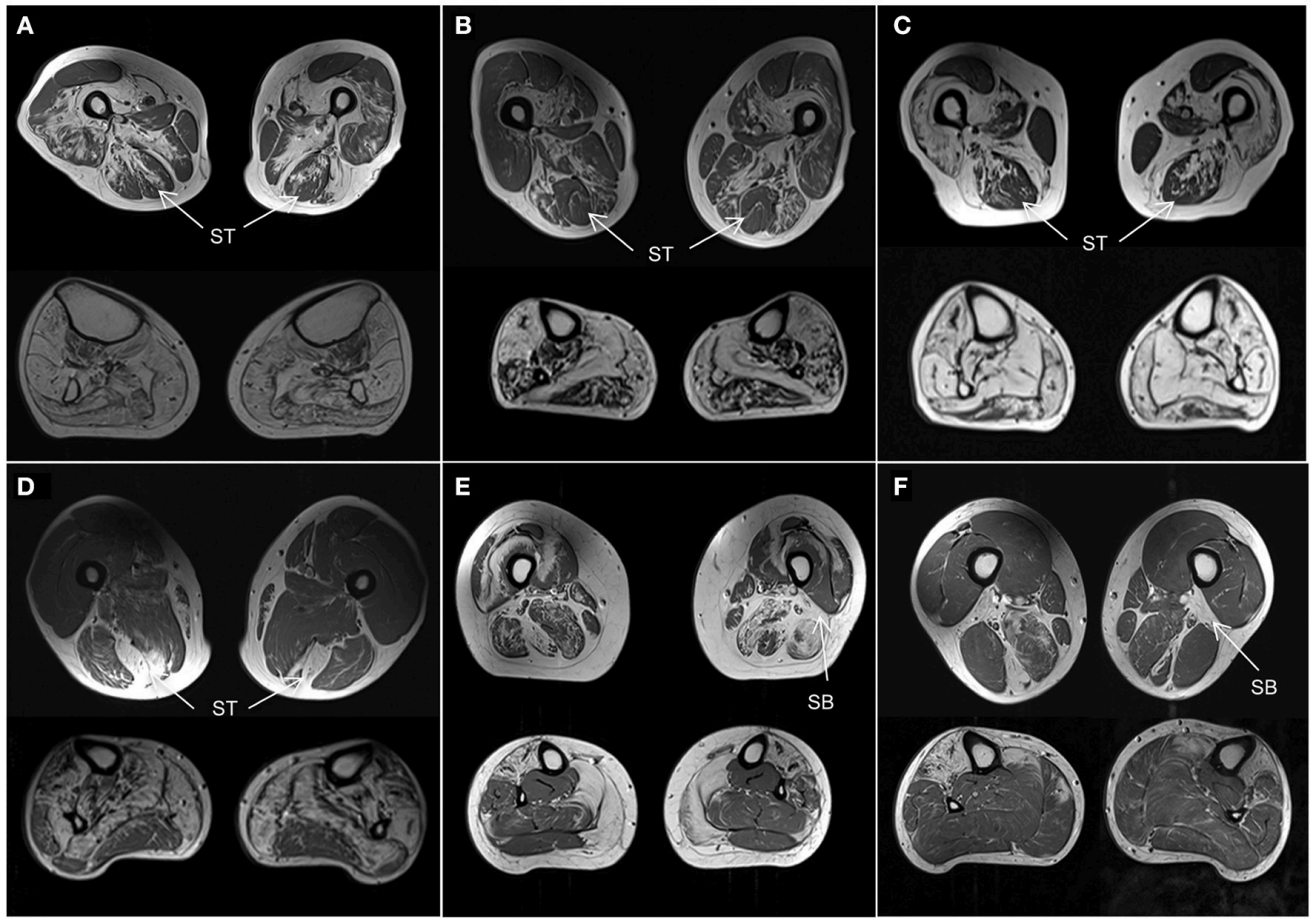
Muscle MRI patterns. Axial T1-weighted images at mid-thigh and mid-calf level in selected subjects. (A–C) Patients with genetically confirmed *MYOT* mutations all correctly identified. They are all characterized by a relative sparing of the semitendinosus (ST) muscle. The vastus intermedius and sartorius muscles are more affected than the gracilis muscle. All calf muscles can be affected; the gastrocnemius lateralis muscle is usually less affected than the medialis. (D) Patient with *TTN* (ID18) mutation. He was not correctly identified as the calf was severely involved without the characteristic pattern. However the selective involvement of semitendinosus (ST) within the thigh is characteristic and could represent a key feature of this disease. (E,F) Patients with *GNE* mutations. One patient (E, ID11) was not correctly identified. The rectus femoris muscles were spared and the vastus lateralis muscles were involved contrary to the reported pattern. However both patients (E,F) revealed severe involvement of short head of biceps femoris (SB) muscles which could represent a key feature of the disease.
